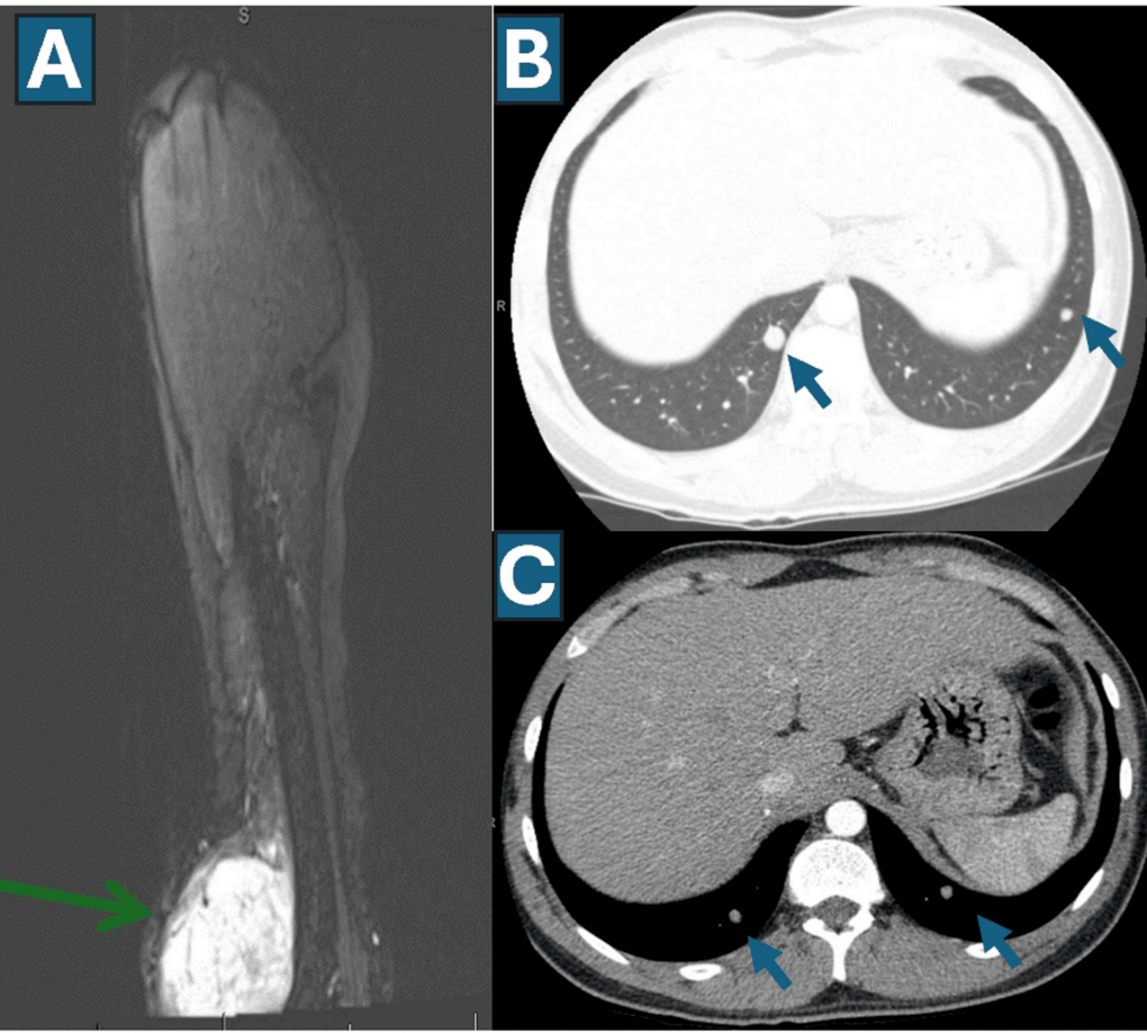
Sequential representative images from the time of diagnosis (A), followed by when lung metastases were first identified (B and C) A. MRI of the left arm showing the mass (blue arrow) arising from the ulna (at initial presentation). B. CT chest with intravenous contrast showing bilateral pulmonary metastases (blue arrows showing two representative lesions). C. Thin slices (1.5 mm) further confirming the findings as in B (blue arrows showing two representative lesions).
